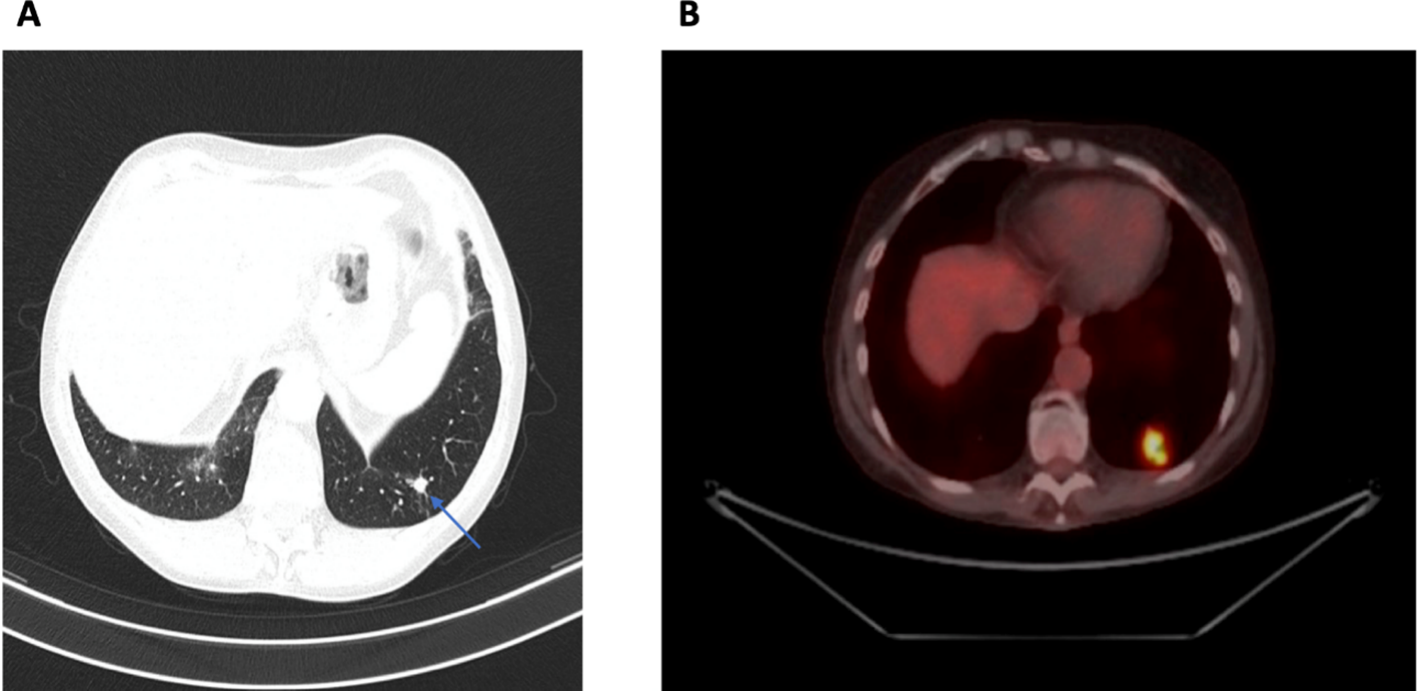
(A) A CT scan of the chest revealed a left lung tumor (blue arrow). (B) FDG-PET/CT examination revealed a polycyclic nodular lesion of the left lung with dimensions of approx. 25x17mm, with a maximum standardized uptake value (SUVmax) of 11.6.
